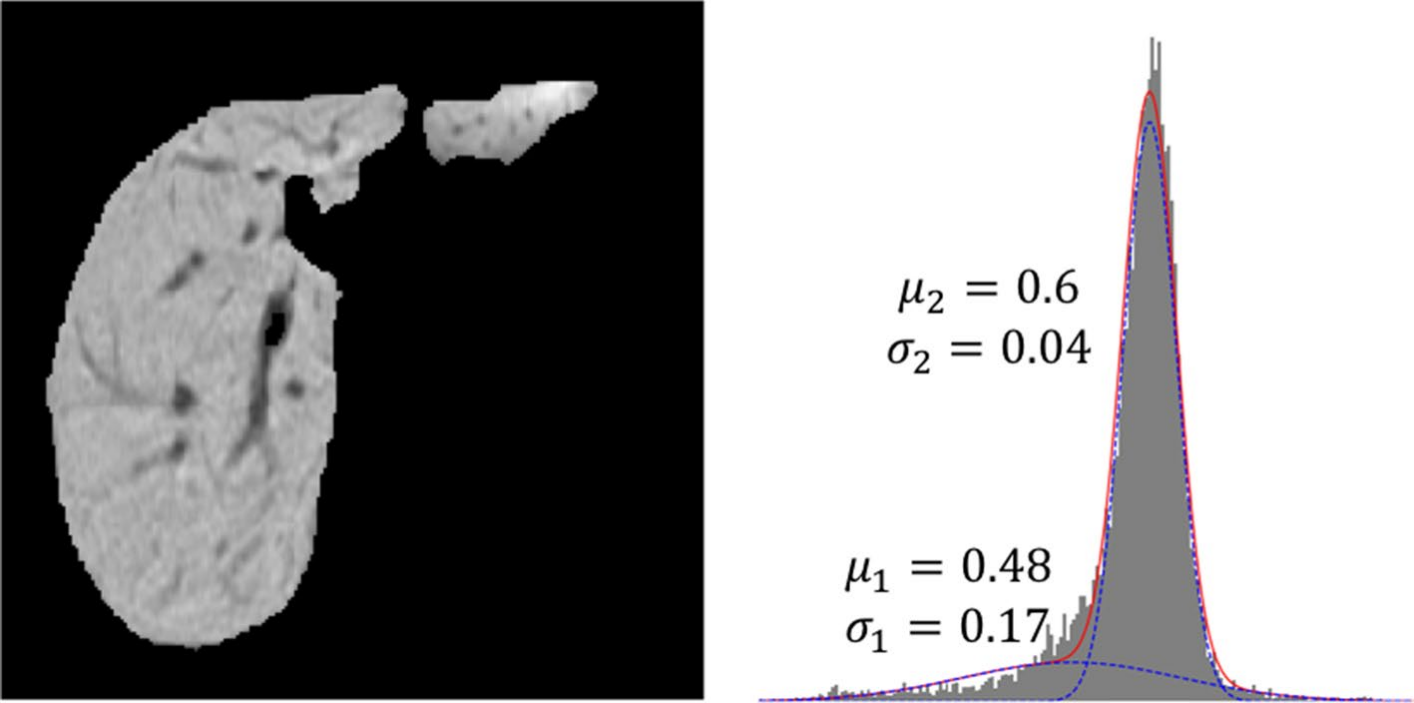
An example of GMM of one liver MR image. The mixture distribution of the image (red line) is well separated by two Gaussian distributions (blue line). The lower curve captures darker blood vessels and the upper curve captures brighter liver background tissue.
